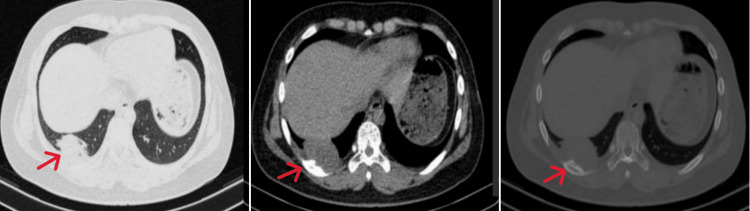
Extraosseous Ewing's sarcoma (EES) chest CT scan of the right chest wall. A. Axial lung window. B. Axial mediastinal window. (A-B) Soft tissue mass is seen in the right lower lung and the posterolateral base of the chest wall. C. Axial bone window. Mild bone destruction is seen near the right ninth rib.
